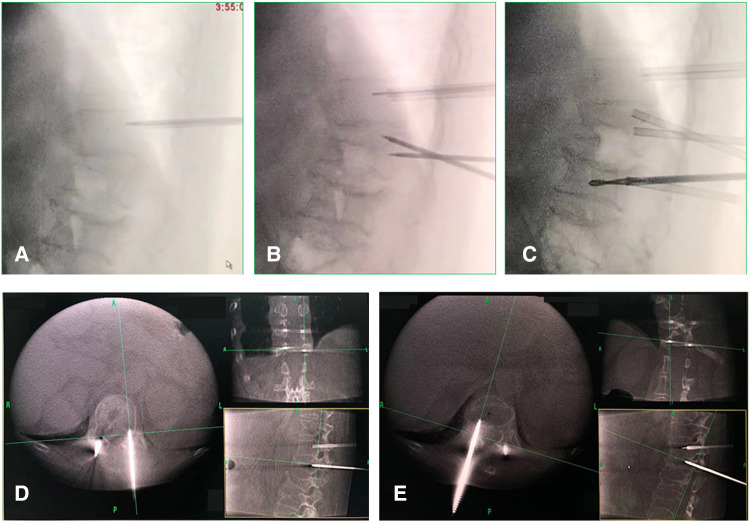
(A,C) The puncture needle was inserted into the target area according to the puncture angle and depth measured before the operation. (B) The other puncture cannula was inserted into the intervertebral disc space adjacent to the ruptured endplate through the transpedicular access. (D,E) Thin-cut CT images were obtained intraoperatively by the O-arm fluoroscopy to confirm puncture needle placement location.
